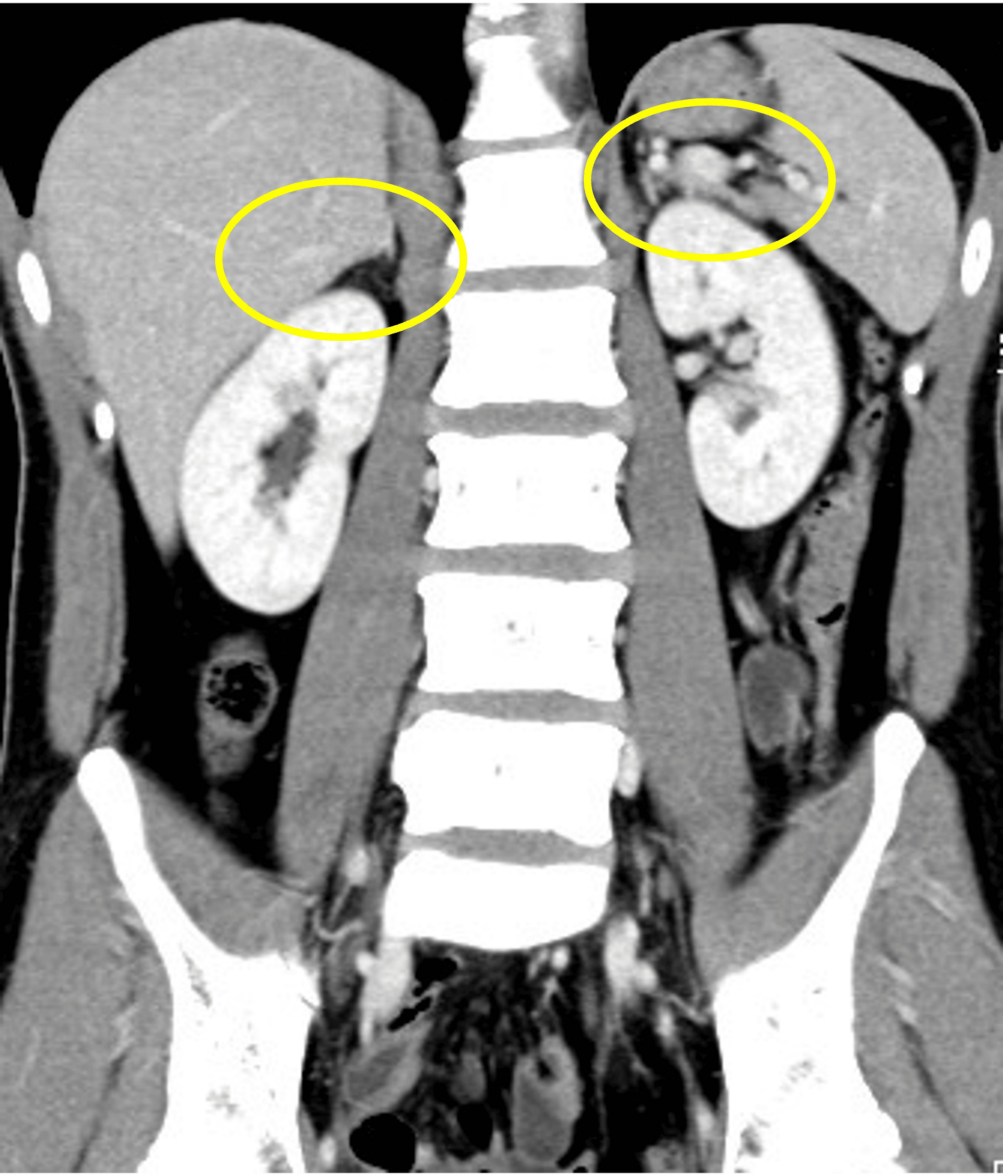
Contrast-enhanced CT scan of the abdomen Coronal view of contrast-enhanced abdominal CT. Both adrenal glands appear atrophic, with no evidence of enlargement or tumor formation.
